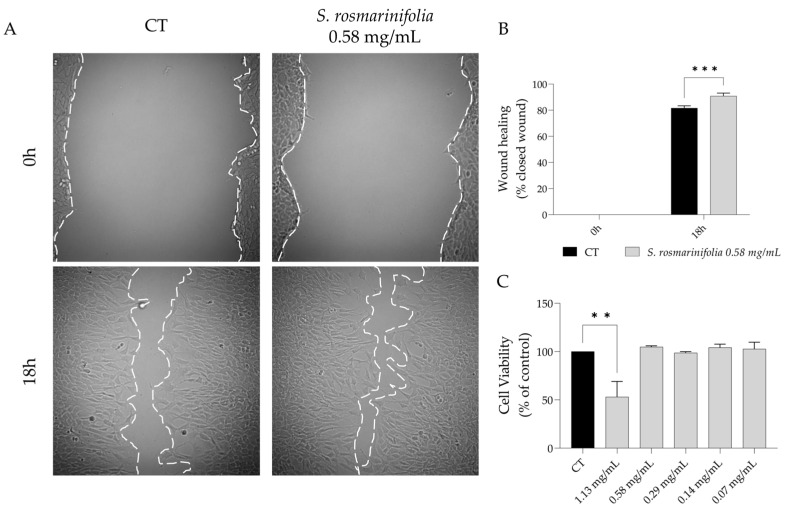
Santolina rosmarinifolia* promoted NIH 3T3 fibroblasts migration using the scratch wound healing assay. (A) Representative bright-field images of NIH 3T3 fibroblasts 0 and 18h post-scratch. White line limits wound edges. (B) Percentage of closed wound at 0 h and 18 h post-scratch. (C) Cell viability of NIH 3T3 fibroblasts after 24 h of treatment with the essential oil. Results show the mean ± SEM of three independent assays made in duplicate.  *p* < 0.01 and * *p* < 0.001 when compared to control (CT).
